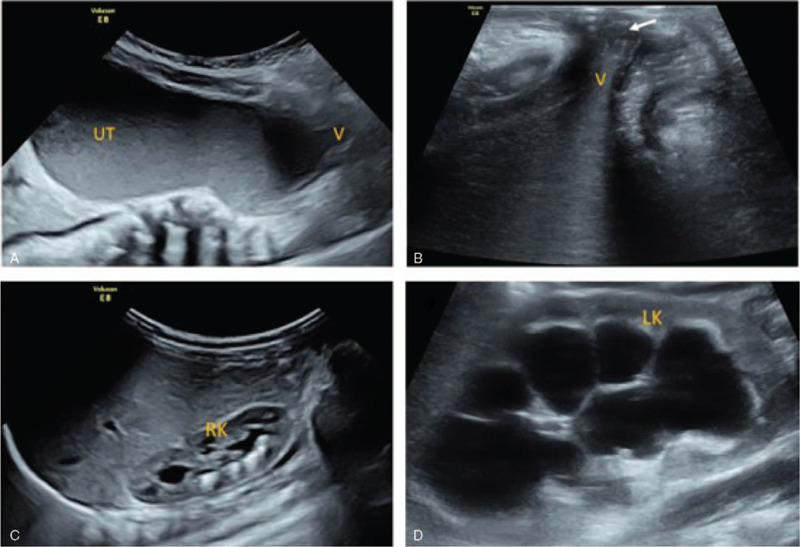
(A) Pelvic sonography showed the cystic mass was significantly enlarged (the bladder was empty). (B) Transperineal high-resolution ultrasound detected the hymen clearly (white arrow). (C) The coronal abdominal sonogram presented mild right hydronephrosis. (D) High-resolution ultrasound showed severe left hydronephrosis. LK = left kidney, RK = right kidney, UT = uterus, V = vagina.
